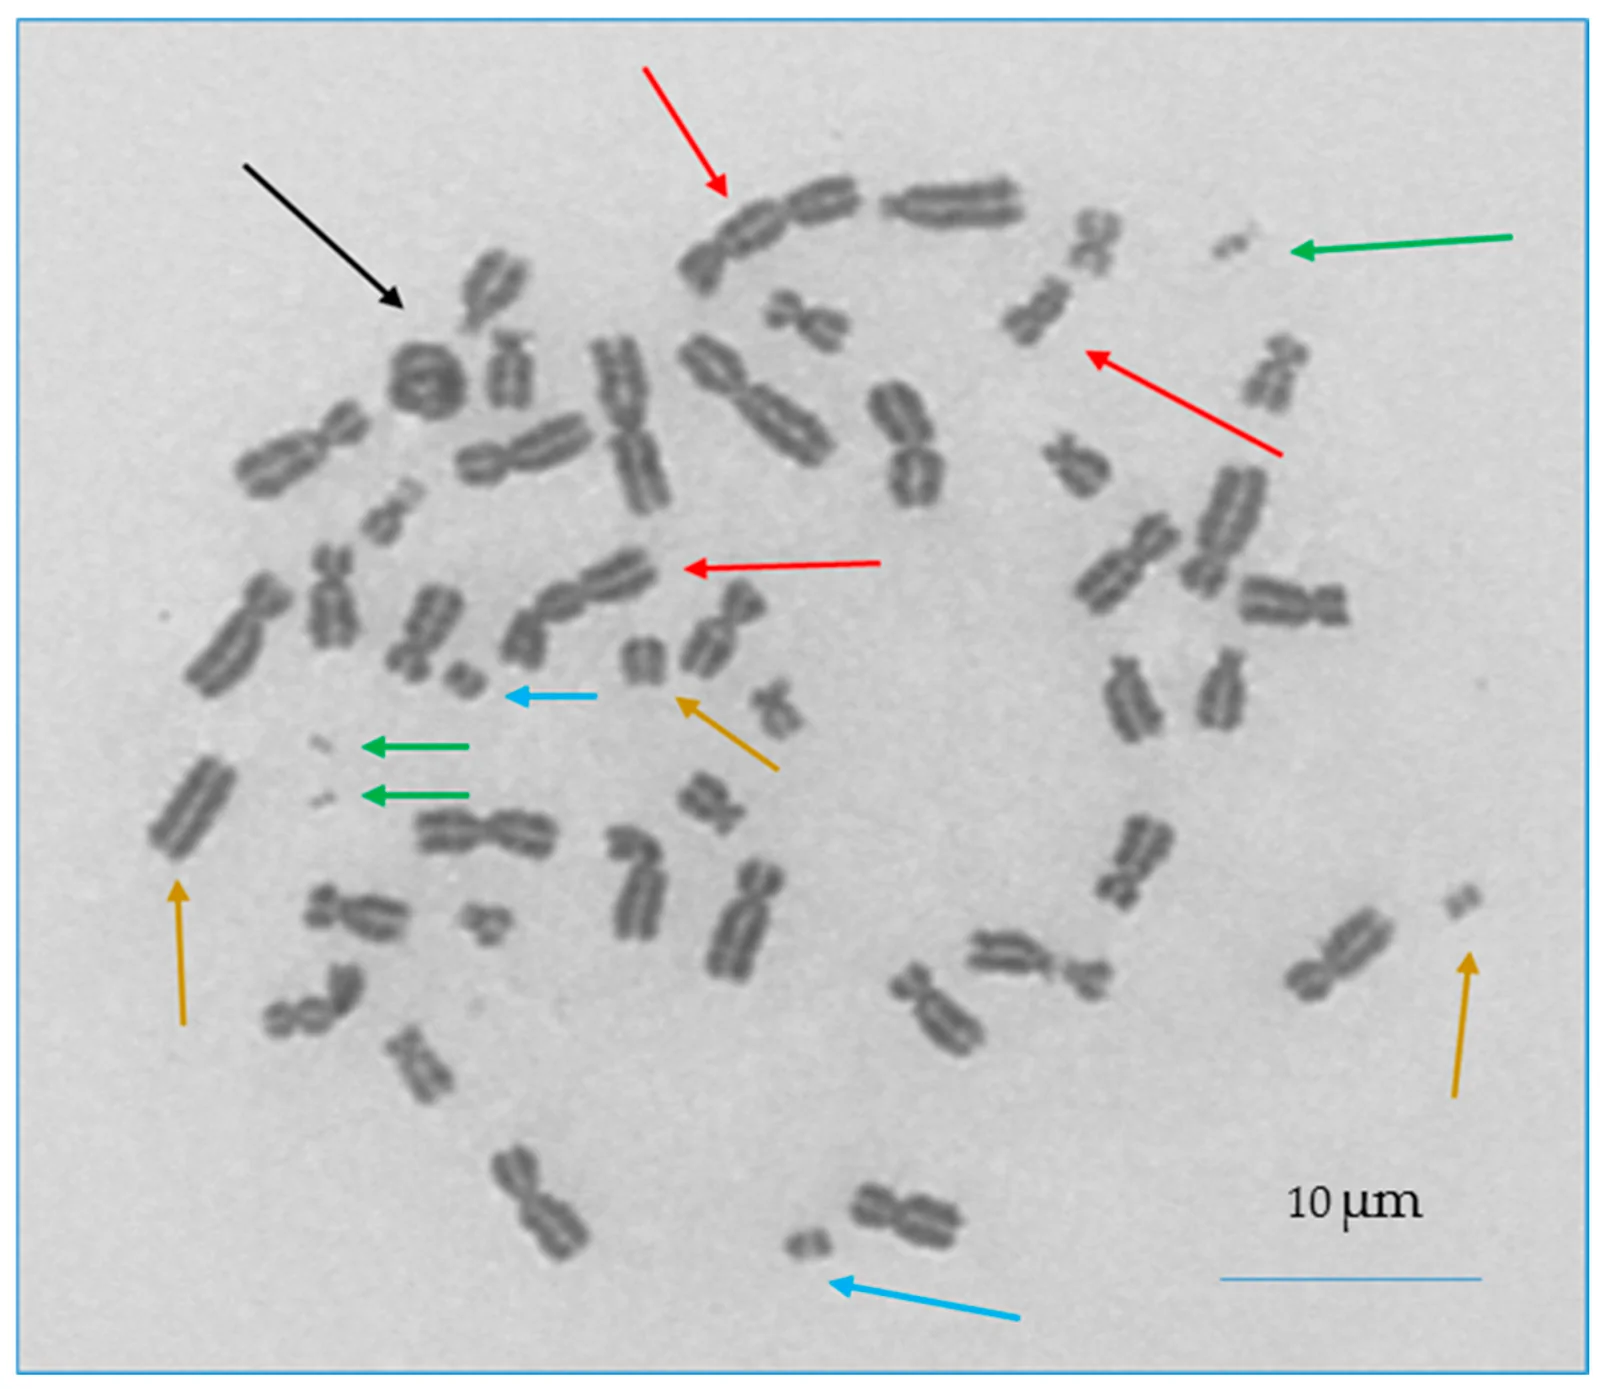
Different chromosomal aberrations in human lymphocytes irradiated with ionizing radiation (X-rays, YXLON portable X-ray flaw detector, dose rate 1.1 Gy/min) [30]. Different types of aberrations are indicated with arrows: red arrows—dicentric chromosomes; black arrow—centric ring. Eight acentric fragments of different shapes and lengths, are indicated with arrows in three colors: green arrows—acentrics resemble double minutes; blue arrows—acentrics resemble acentric rings; brown arrows—acentrics with a pronounced length.
